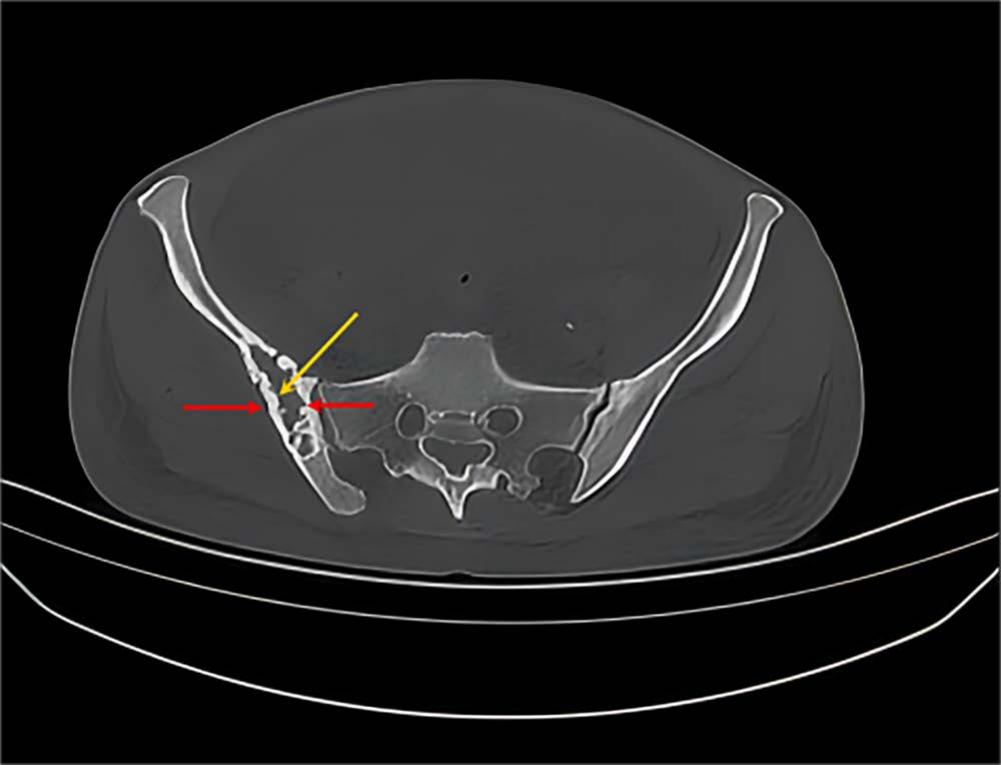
Computed tomography presented a large lytic lesion (yellow arrow), which was surrounded by the sclerotic rims (red arrows) on the right iliac bone.
